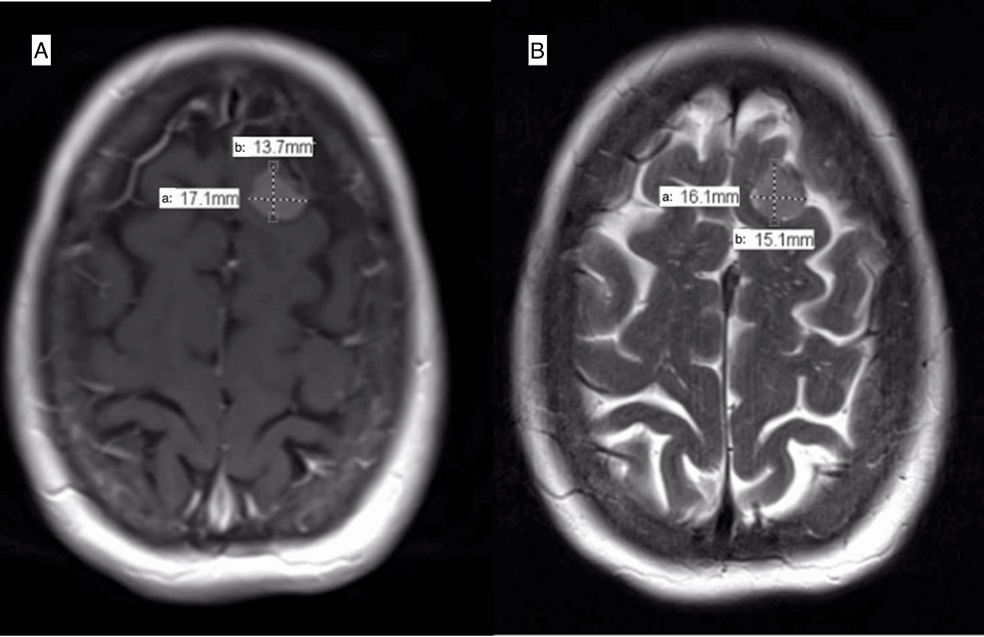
Measuring axial (a) and perpendicular (b) dimension diameters of meningioma T1 post-contrast (A) and T2 FSE (B) images using Philips IntelliSpace Radiology. (A) T1 post-contrast MRI of a meningioma measuring 17.1 mm in the axial (a) dimension and 13.7 mm in its perpendicular (b) dimension; (B) T2 FSE MRI of a meningioma measuring 16.1 mm in the axial (a) dimension and 15.1 mm in its perpendicular (b) dimension. FSE: fast spin echo; MRI: magnetic resonance imaging
